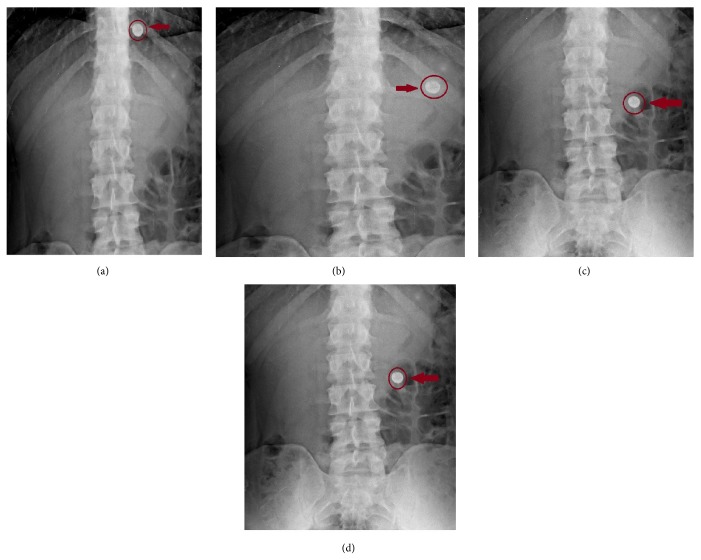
X-ray of formulation F5 after time interval: (a) 0.5 hrs; (b) 2 hrs; (c) 4 hrs; and (d) 6 hrs.
